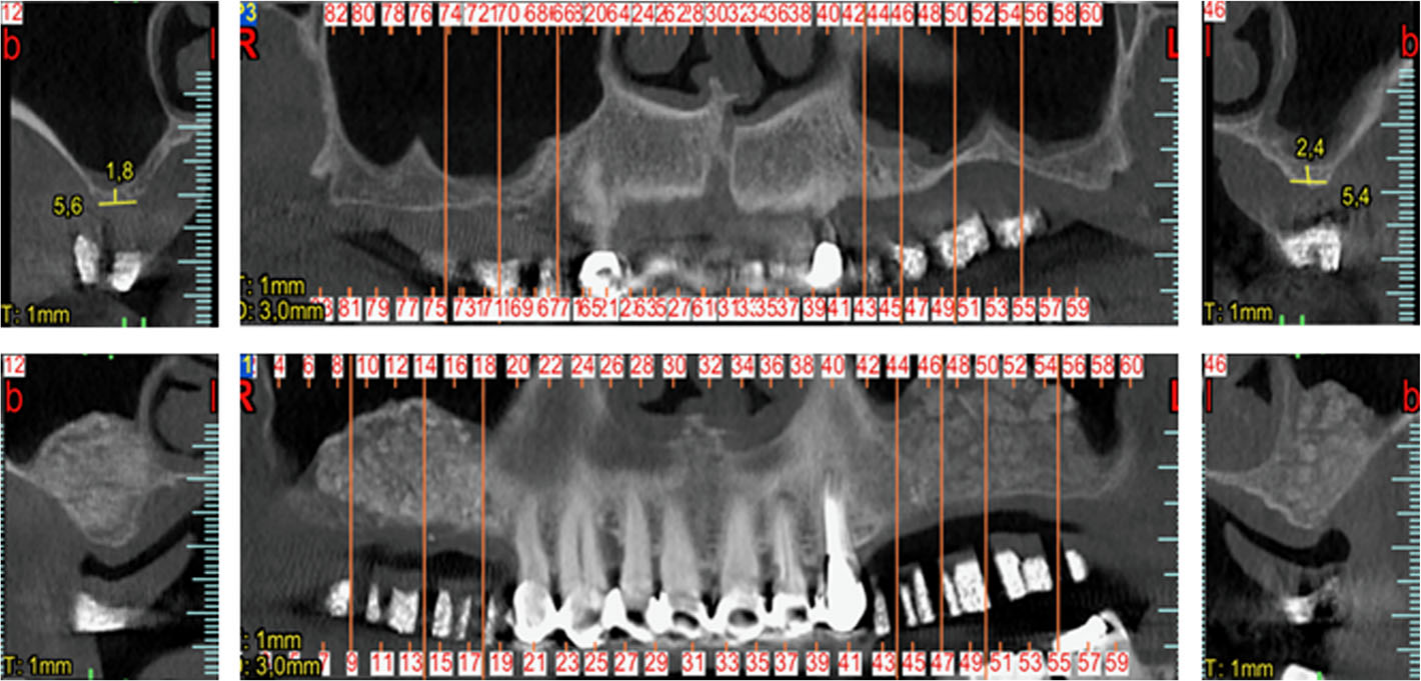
CBCT scans of the bilateral case depicted in Fig. 3, before (top) and after (bottom) the sinus augmentation surgery
